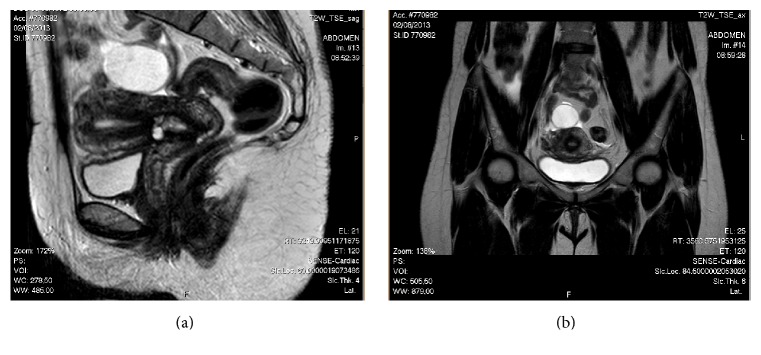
MRI: T2-weighted imaging. (a) Sagittal view. (b) Coronal view: left ovarian cyst.
